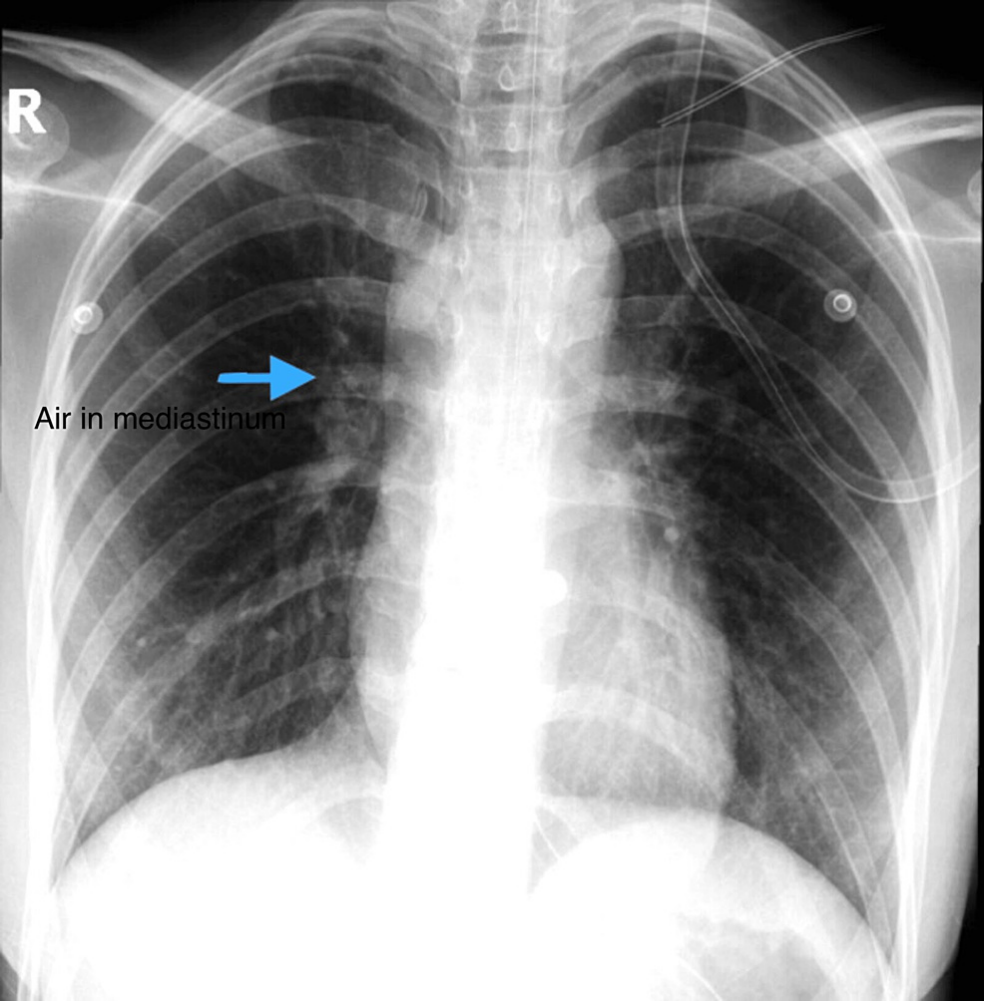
Chest X-ray shows air in the mediastinum.
